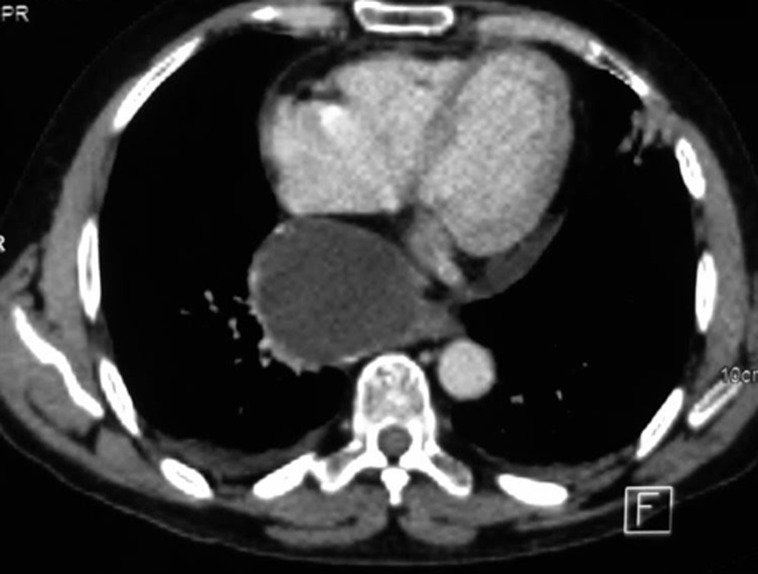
chest CT-scan showing pleuropericardial cyst with 79 x 66 mm at diameter
